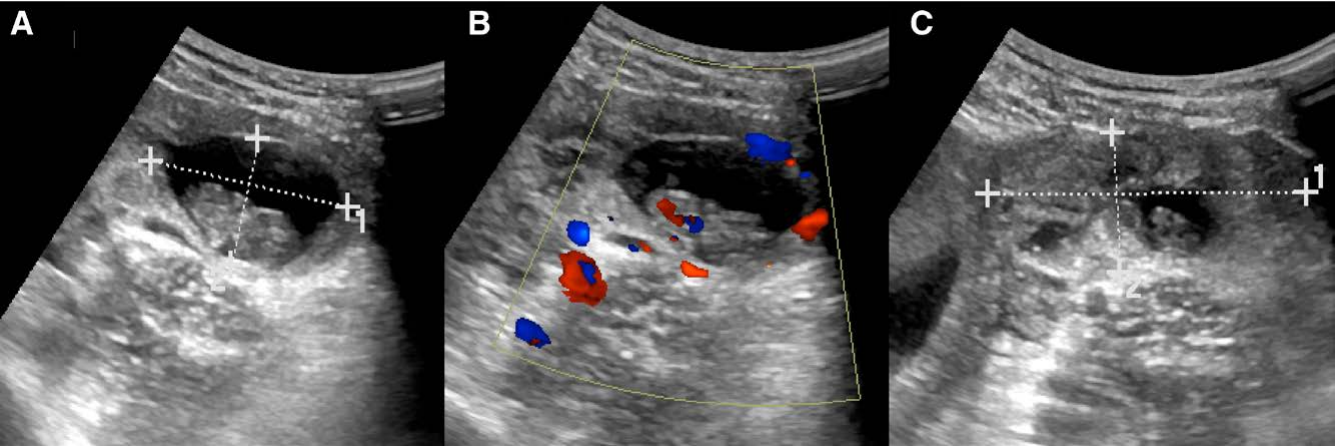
TAS results on day 66 of amenorrhea. (A) Showing EP in the left region, with a gestational sac size of 38 × 24 × 26 mm. (B) CDFI showing primitive cardiac activity in the embryonic pole of the left EP. (C) Embryonic pole size of 25 mm in length. CDFI = color doppler flow imaging, EP = ectopic pregnancy, TAS = transabdominal sonography.
